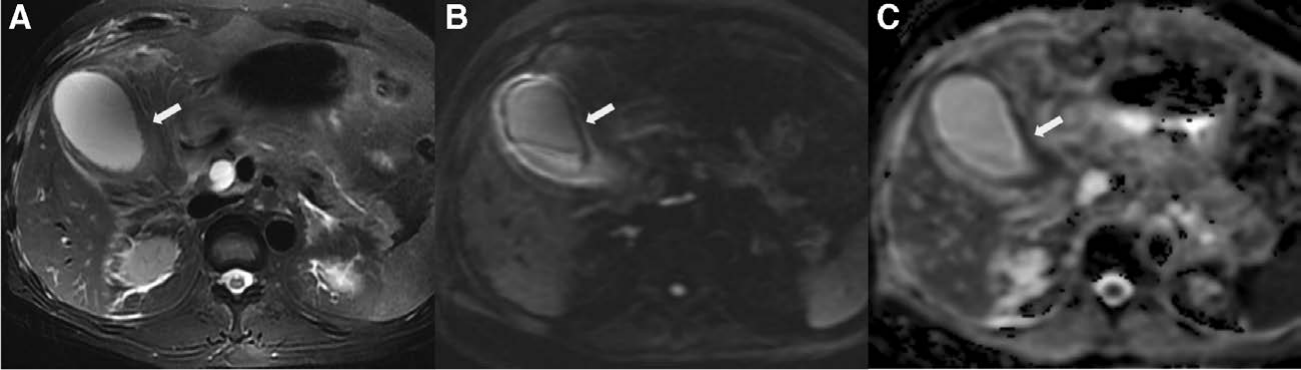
Acute gangrenous cholecystitis in a 49-year-old male. (a) Diffuse hypointensity of thickening (arrow) was exhibited in the thickened GB wall on T2WI with fat saturation image, without layered pattern (type 4); The inner wall appeared somewhat papillary change due to mucosal irregularity; (b) Diffuse hyperintensity with layered pattern on DWI images was shown (arrow); (c) The layered pattern was observed on ADC map image, with hypointensity (arrow) in the neck of GB (type 2). DWI = diffusion-weighted imaging, GB = gallbladder, T2WI = T2-wighted imaging.
